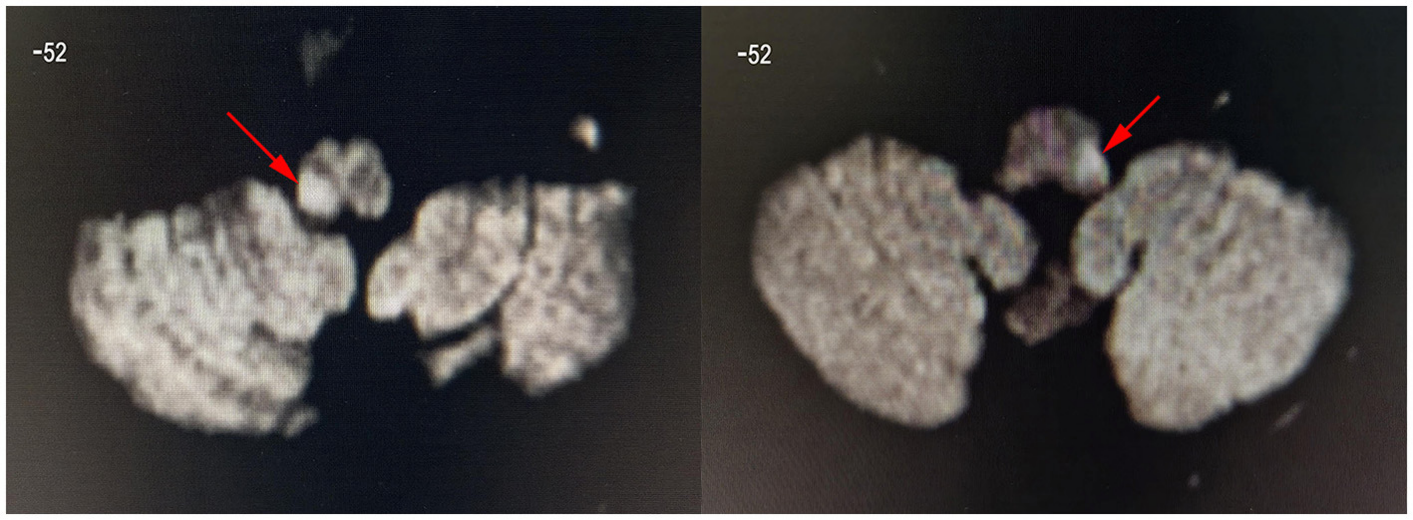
Diffusion-weighted images showing dorsolateral medulla infarction (arrow). These are the DWI images of patient 8 (left image, lesion located in the right medulla oblongata) and patient 1 (right image, lesion located in the left medulla oblongata). The z-value of the coordinate for the slice position is −52. All lesions were located in the left or right side of dorsolateral medulla oblongata.
